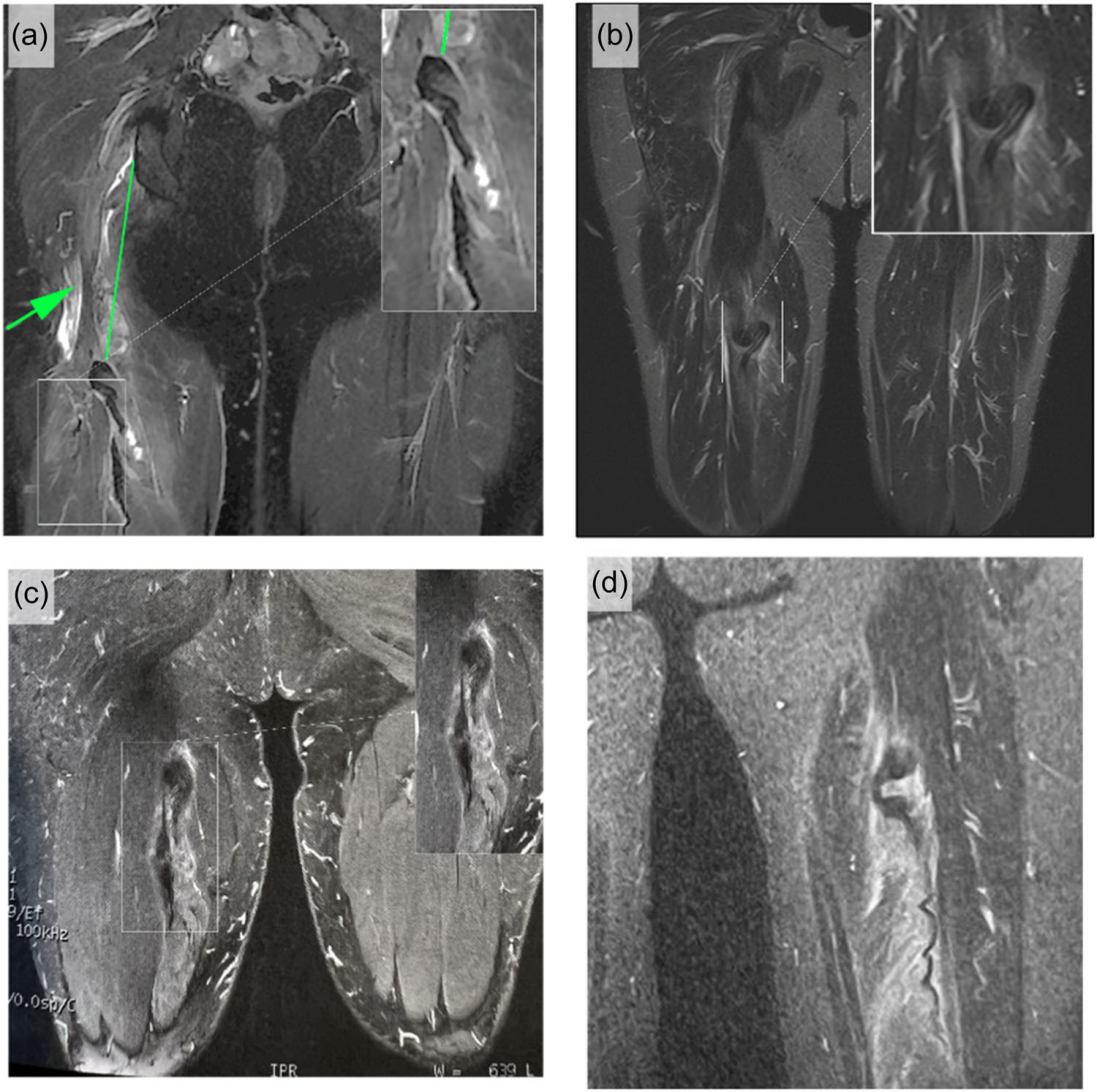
Cobra sign as identified in four patients with isolated semimembranosus rupture. (a) Coronal T2‐weighted MRI of a 50‐year‐old female patient presenting with a domestic injury due to a wide stretch (fall or slip), showing the classic cobra sign with 11 cm retraction. (b) Coronal T2‐weighted MRI of a 52.93‐year‐old male patient presenting with a sports‐related injury due to a wide stretch (fall or slip), showing the classic cobra sign with 13.3 cm retraction. (c) Coronal T2‐weighted MRI of a 48‐year‐old male patient presenting with a sports‐related injury due to a sudden acceleration (rapid start), showing the classic cobra sign with 12 cm retraction. (d) Coronal T2‐weighted MRI of a 55‐year‐old female patient presenting with a sports‐related injury due to a sharp deceleration, showing the classic cobra sign with 9 cm retraction.
